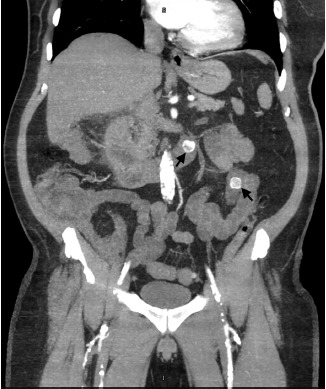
Computed tomography angiography (coronal view) demonstrating two rim-calcified gallstones within the proximal jejunum (black arrows).
